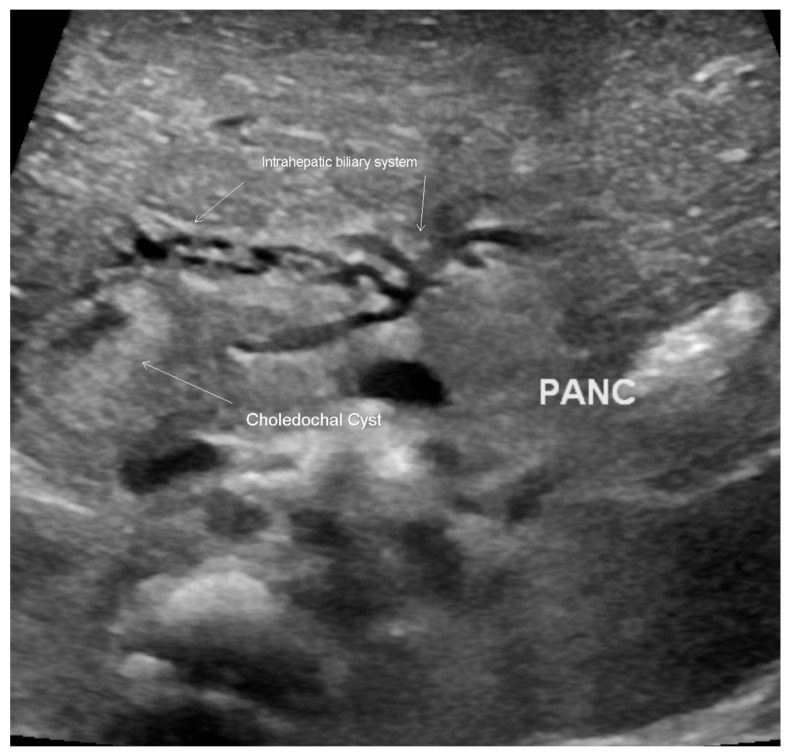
Ultrasound scan of the abdomen, revealing a choledochal cyst with dilatation of the intrahepatic biliary system.
